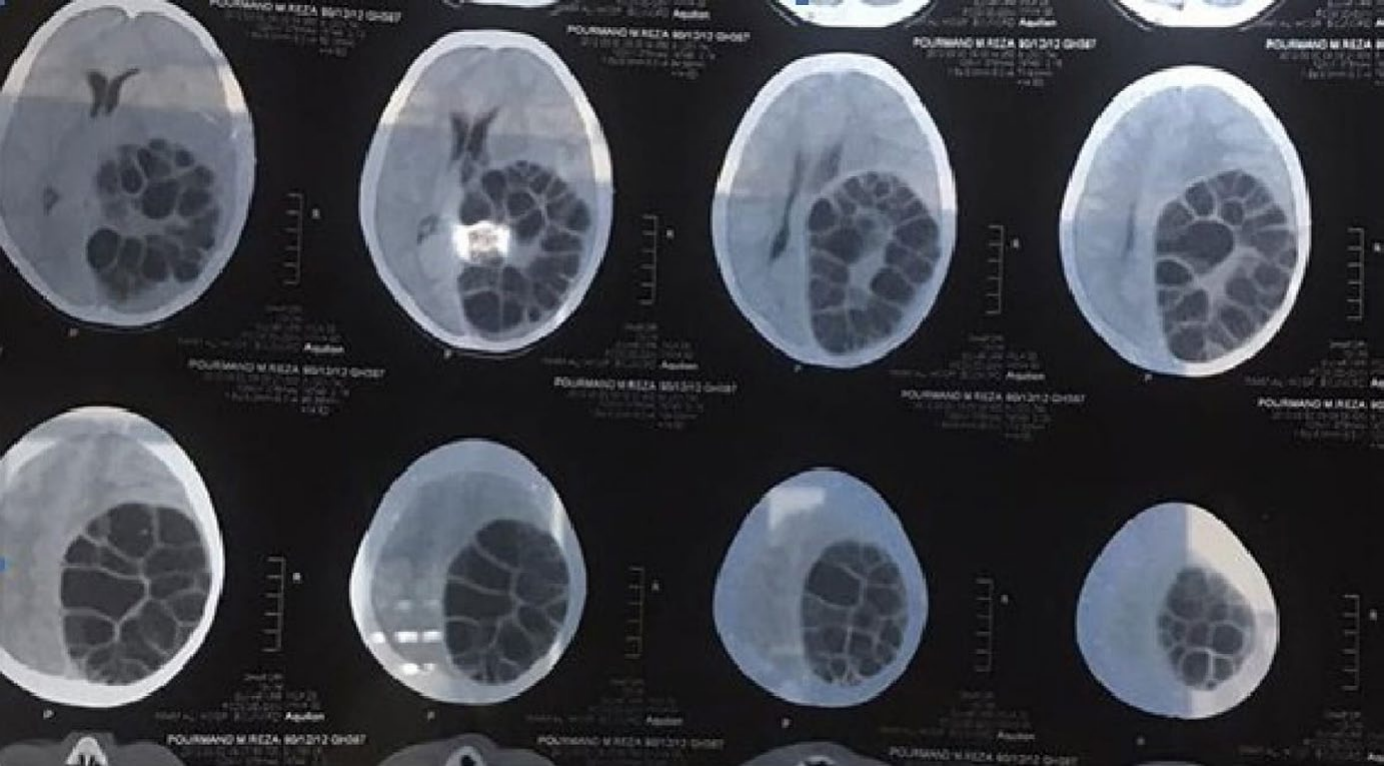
Preoperation CT scan revealed a large cystic left parietal and occipital lobe, paraventricular area
